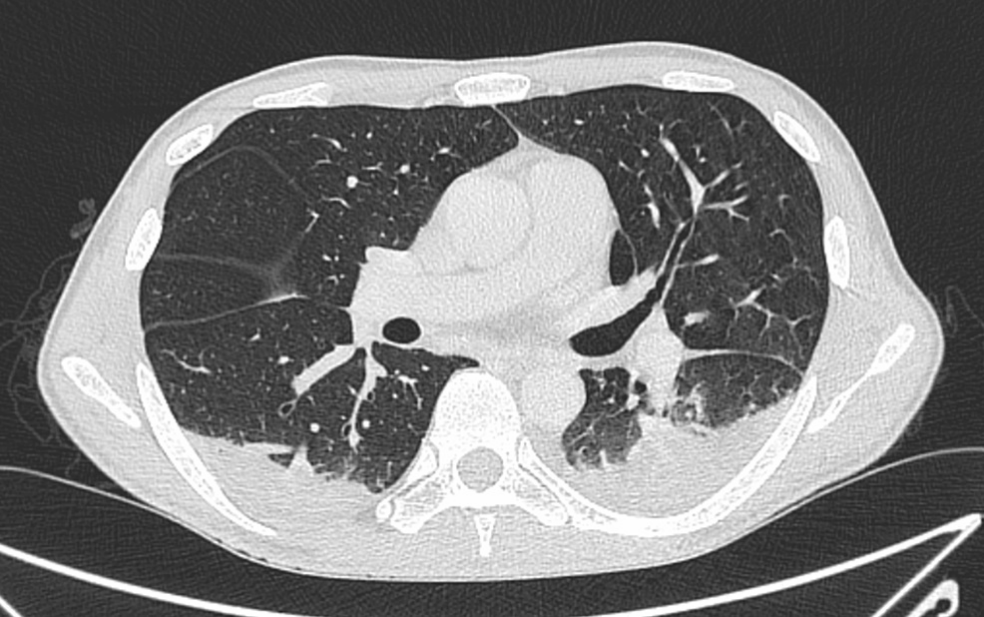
Pulmonary CT scan on day 16 after admission: SARS-COV-2 bilateral pneumonia
